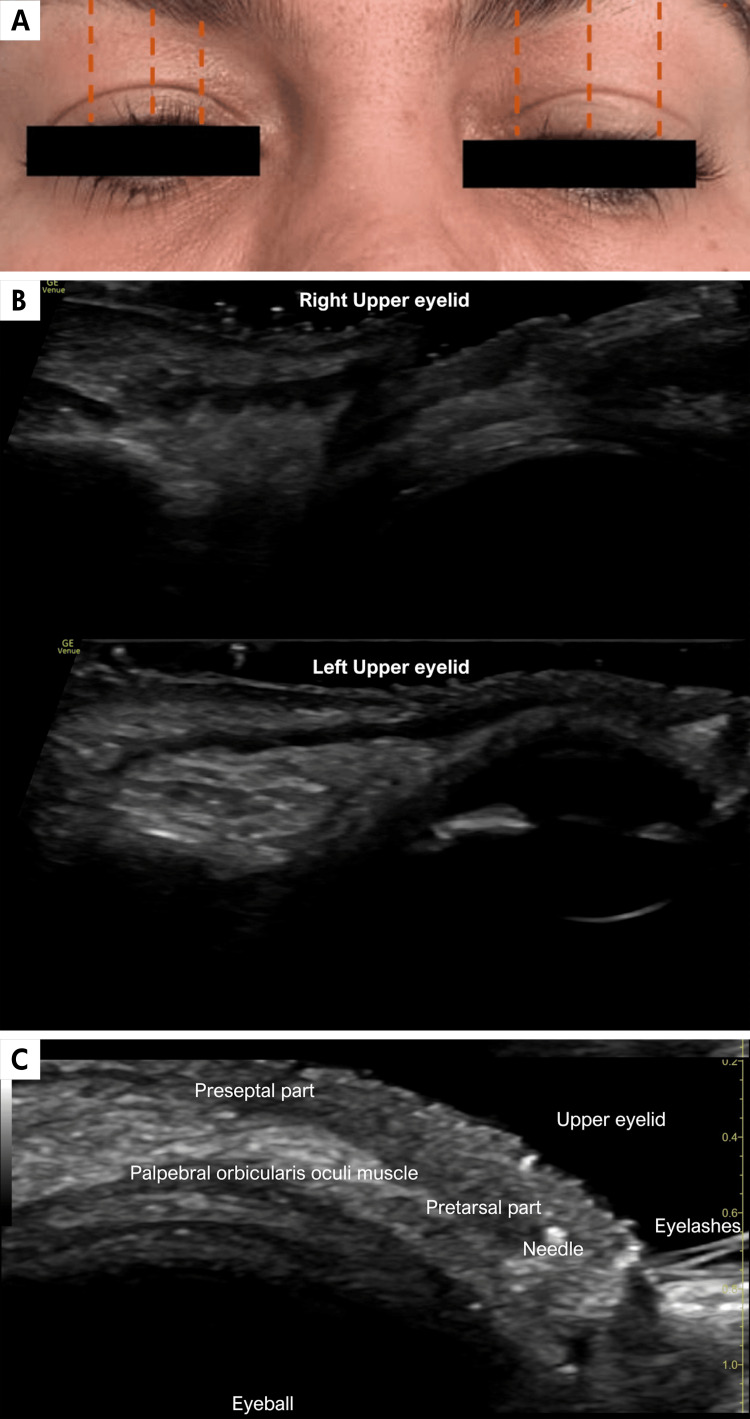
Ptosis seen with high-resolution gray-scale ultrasound. A. Clinical image showing asymmetry in the length of the orange lines, demonstrating left ptosis. B. High-resolution ultrasound with a linear probe (18 MHz) revealing incomplete contraction of the orbicularis oculi muscle in the left eyelid secondary to an incorrect technique in the botulinum toxin injection on the left side. Normal and complete contraction is observed on the right side. C. Ultrasound-guided injection of botulinum toxin into the pretarsal part of the orbicularis oculi muscle in the left upper eyelid for treatment.
